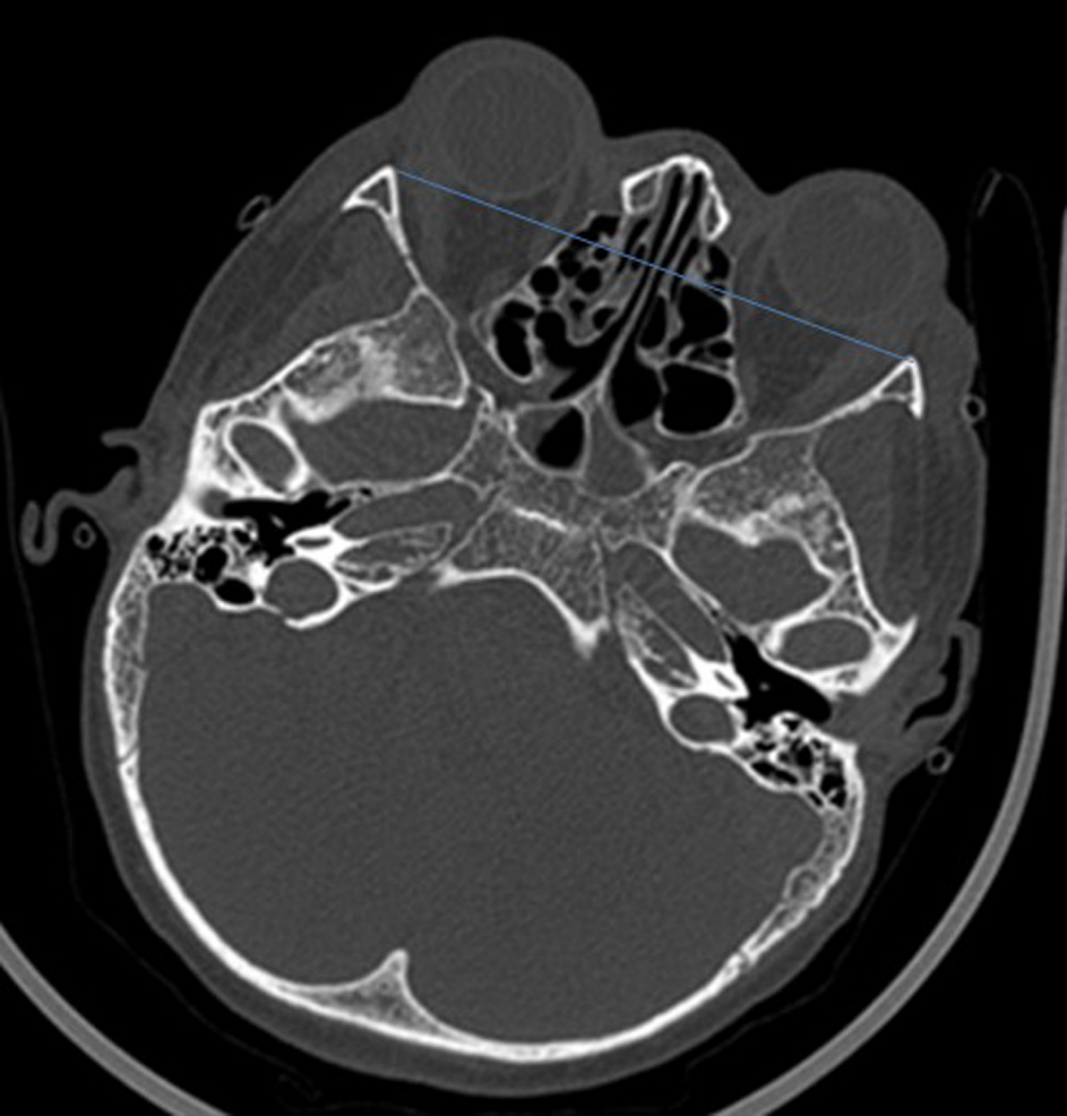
Oculocerebral CT scan with bone window: exophthalmia stage 3, with the absence of abnormalities in bone structure
